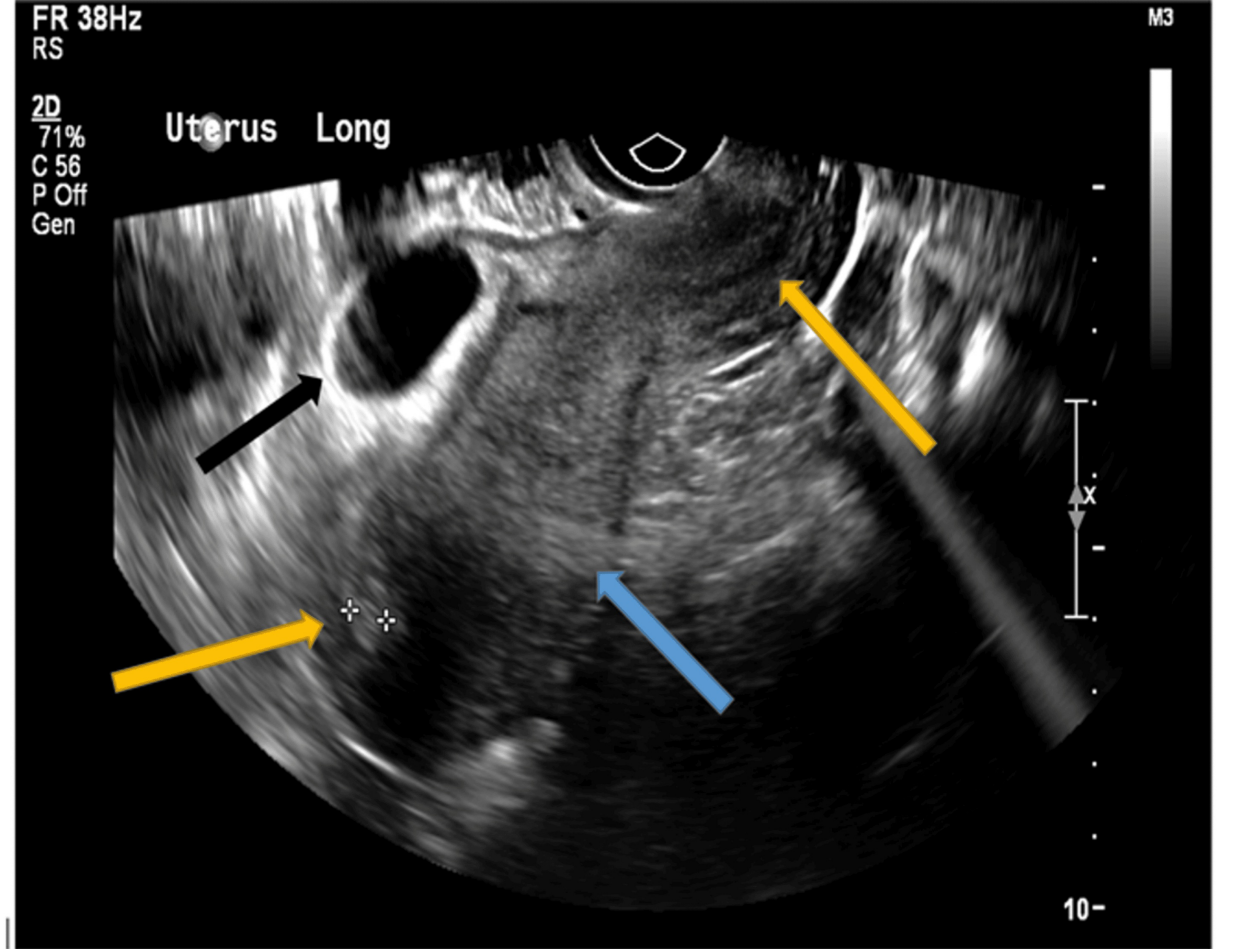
Longitudinal transvaginal ultrasound showing an ectopic cesarean scar pregnancy The black arrow indicates the thinning of the anterior aspect of the myometrium. The blue arrow indicates the normal thickness of the posterior myometrial wall. The yellow arrows indicate empty endometrium.
